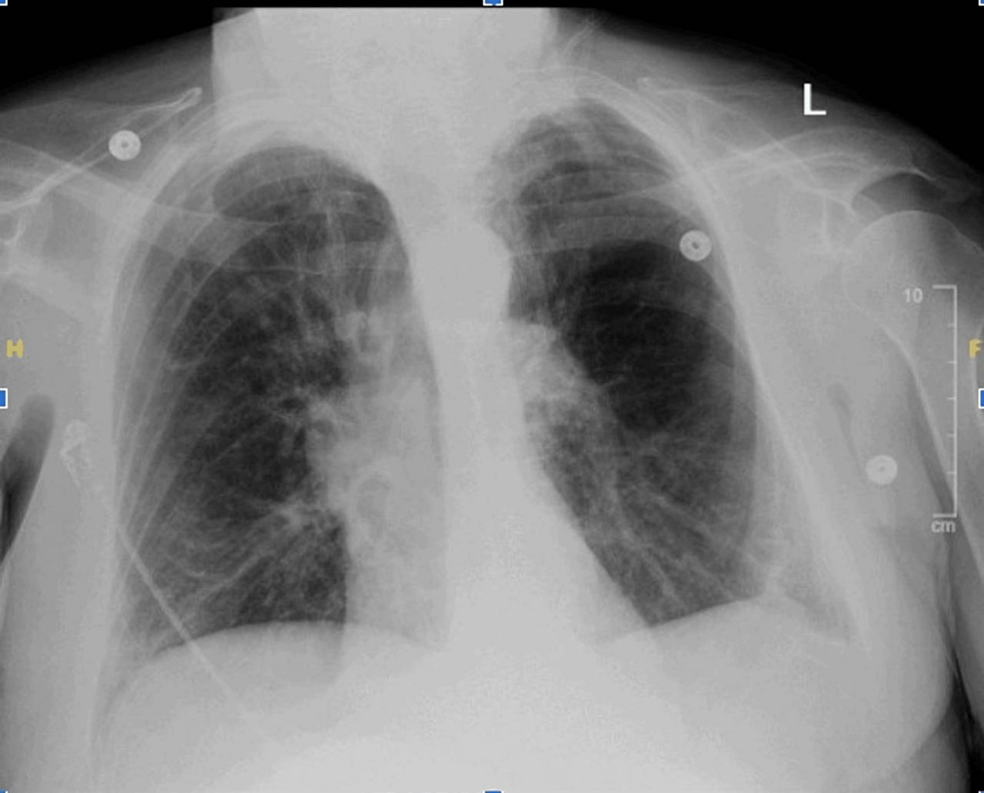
Chest X-ray showing prominent markings present in both lungs, with a small region of atelectasis/infiltrate in the left lower lobe.
